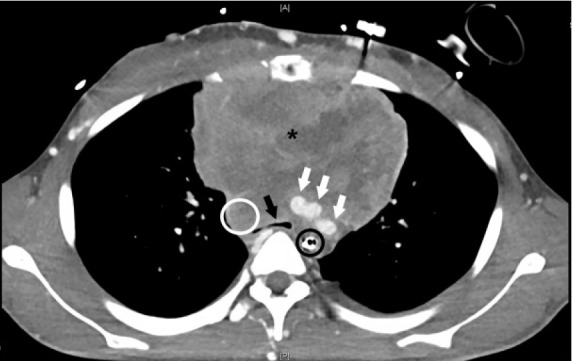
Axial computed tomography with contrast image of the chest. Contrast within the vessels appears white. A heterogeneously enhancing mass is seen occupying the anterior mediastinum (asterisk). There is significant compression of the trachea (black arrow) at the level of the carina, which is smaller in diameter than the adjacent orogastric tube (black circle). White arrows indicate brachiocephalic, left carotid, and left subclavian vessels just superior to their origins from the aortic arch. The superior vena cava (which should be in the vicinity of the white circle) is conspicuously absent.
